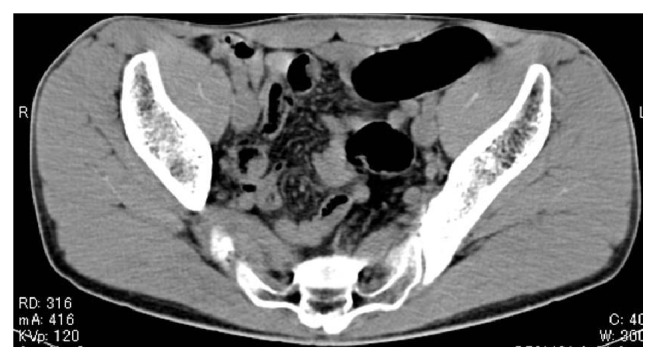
Initial computed tomography image. The image shows only normal gas distribution in the gastrointestinal tract; there is no indication of a foreign body.
